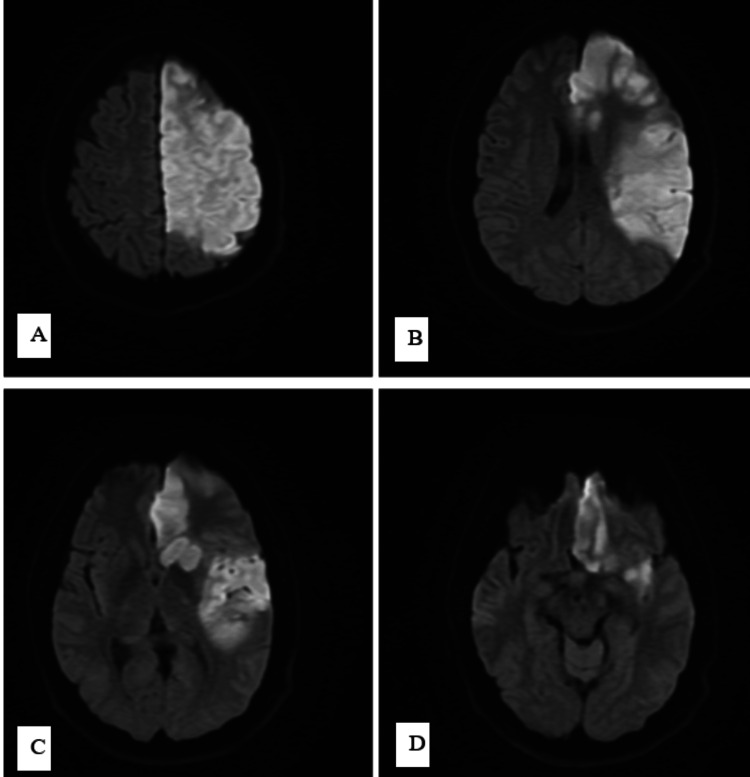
Acute infarct distribution on diffusion-weighted MRI (hospital day 4) Axial diffusion-weighted images at four levels demonstrate extensive left hemispheric acute infarction with restricted diffusion. The superior slices (A, B) show involvement of the medial/parasagittal frontal cortex, consistent with a left anterior cerebral artery (ACA)-territory component, while the more inferior slices (C, D) demonstrate left middle cerebral artery (MCA)-territory infarction involving the insula, operculum, and deep gray structures.
